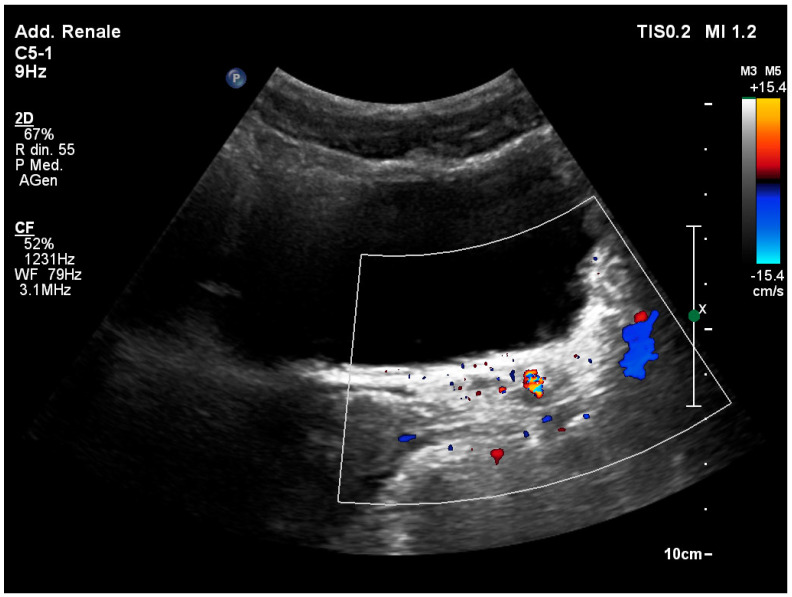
A twinkling effect is produced by a calculus located near the left ureteral meatus. The artifact is seen as a turbulent flow immediately after the calculation, as determined by the color Doppler.
